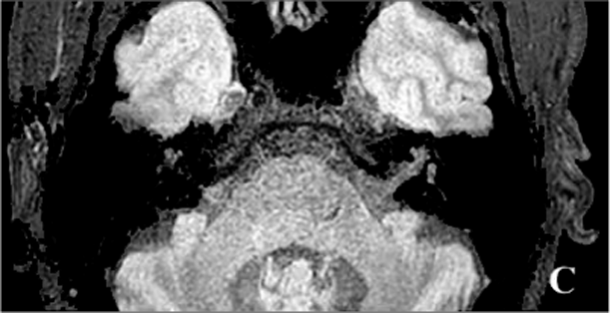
In April 2020, the signals of the left vestibular and vestibular aqueduct remained higher than that in the contralateral in the 3D-T2-FLAIR sequence. Nevertheless, the signal differences decreased (C), indicating that inflammatory lesions had subsided.
